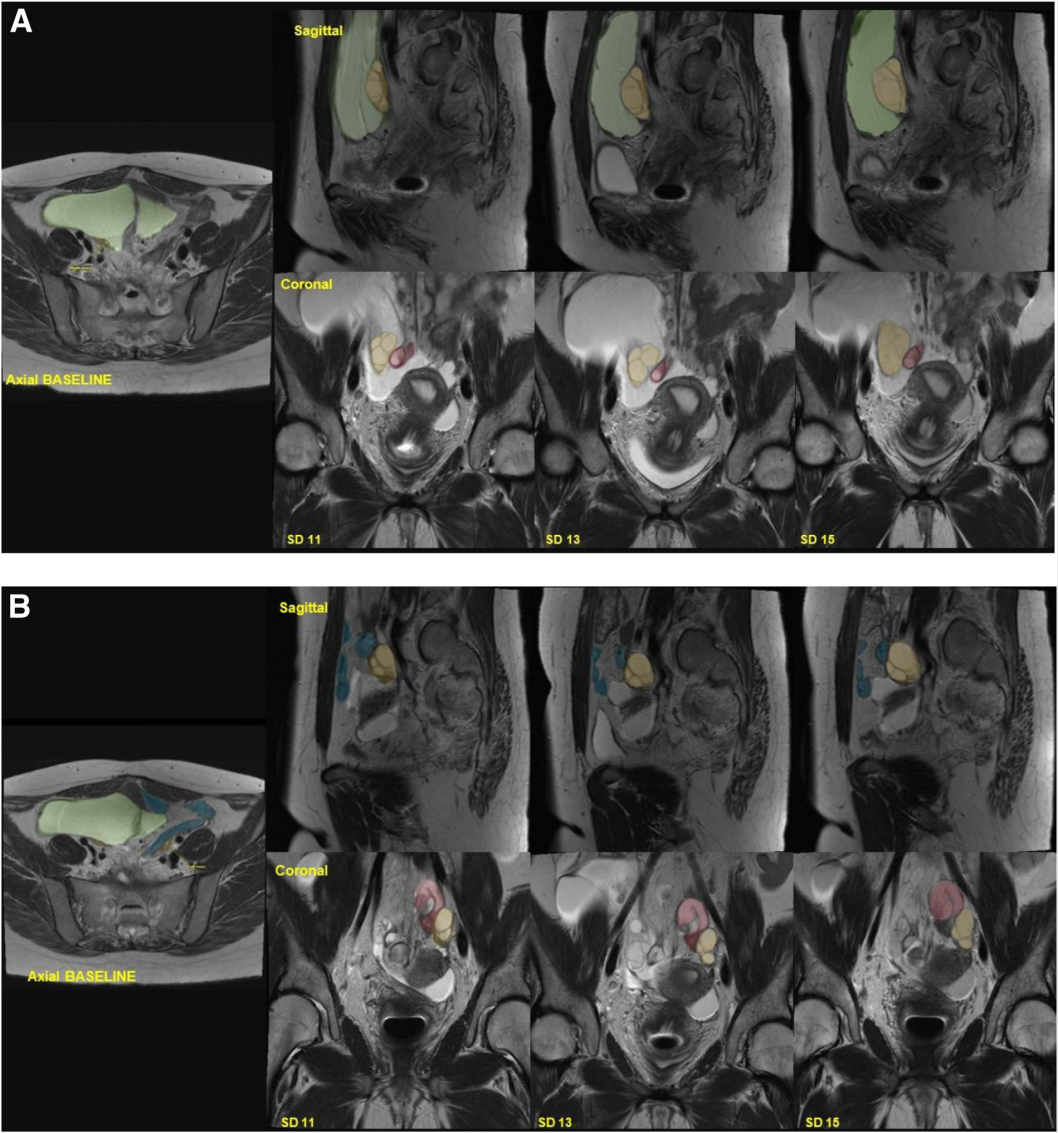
Daily monitoring and evaluation of right ovarian stimulation by using magnetic resonance imaging. (A) Right ovary. (B) Left ovary. Green shading indicates the inclusion cyst, blue shading represents the bowel segments, yellow shading denotes the ovaries, and red shading highlights the presence of a hydrosalpinx. SD = stimulation day.
